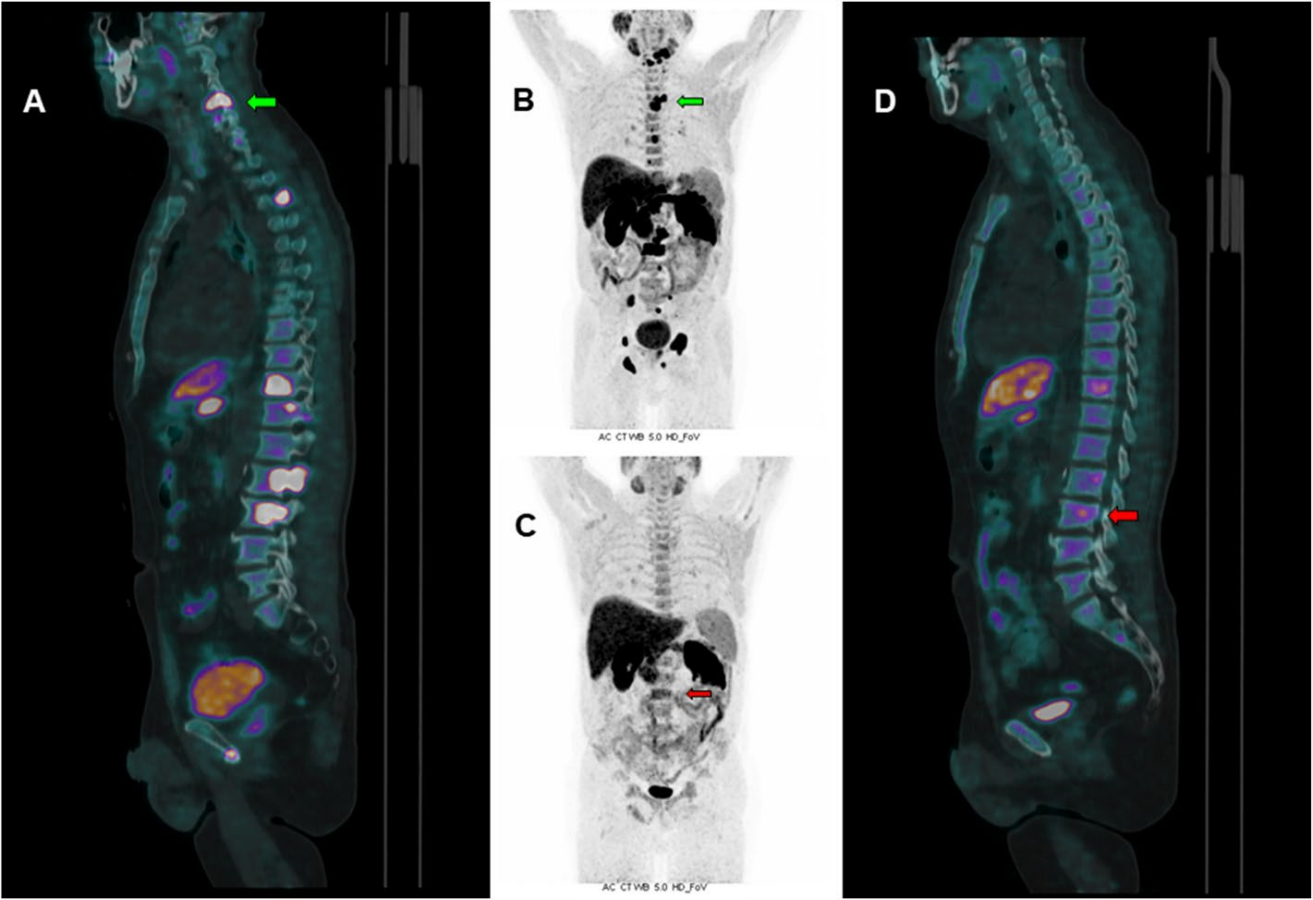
Baseline [18F]F-choline PET/CT scan (A sagittal fused image, B maximum intensity projection) showed prostatic increased uptake and several bone uptakes (i.e. SUVmax 25.5 on L3), suggestive for metastasis (green arrow). [18F]F-choline PET/CT scan, performed about 7 months later, with ongoing ADT plus docetaxel therapy (C maximum intensity projection; D sagittal fused image) showed only minimal residual vertebral uptake (red arrow, SUVmax 10.3 on L3)
